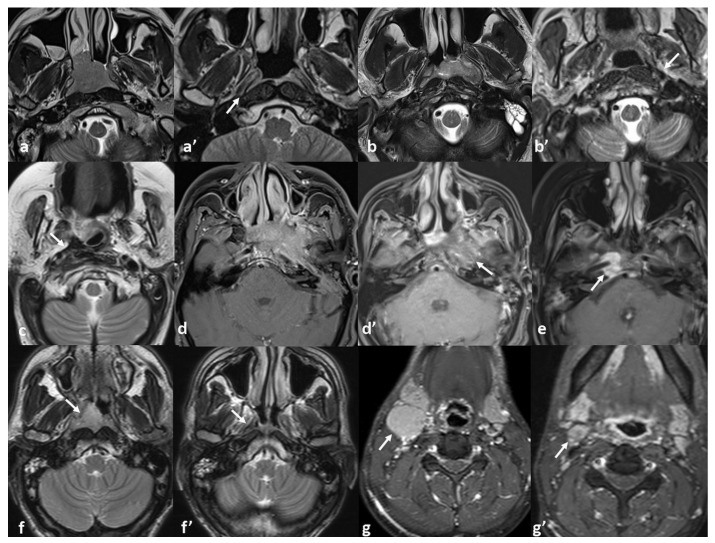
Pre- and post-treatment MRI findings demonstrating response patterns. Original letters, e.g., (a), indicate pre-treatment images; apostrophes, e.g., (a’), indicate corresponding post-treatment images. Axial TSE-Dixon T2WIs (a–c,f) and post-contrast fat-saturated axial TSE-Dixon T1WIs (d,e,g) are shown. (a,a’) Complete response with no residual or post-treatment changes. (b,b’) Linear fibrotic changes at the original tumor site (arrow). (c) Bulky fibrosis obliterating fat planes, without intermediate signal or soft tissue component (arrow). (d,d’) Heterogeneous signal with subtle enhancement, without soft tissue residue (arrow). (e) Residual enhancing soft tissue at the foramen lacerum (arrow). (f,f’) Complete local response (arrow) with (g,g’) residual level IIa lymph node (arrow).
